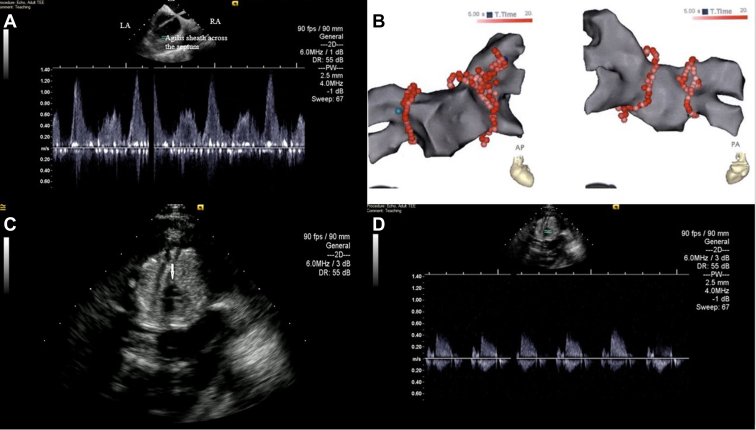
A: Intracardiac echocardiography (ICE) image of Agilis sheath (Abbott) just after transseptal puncture in the plane of left pulmonary veins as evidenced by pulmonary vein pulse vein Doppler images. LA = left atrium; RA = right atrium. B: Pulmonary vein isolation lesion set in anteroposterior (AP) (*left*) and posteroanterior (*right*) views; additional lesions were required to left superior pulmonary vein over the ridge to achieve complete isolation, as seen in AP view. C: ICE imaging of the fetus. From the initial view of the fetus in the long axis, clockwise rotation of the ICE probe revealed a short-axis view with the fetal cardiac atrioventricular (AV) valve (*white arrow*). D: Fetal AV valve Doppler. In the upper half of the image note the pulse wave Doppler cursor at the AV valve. In the lower half of the image note the sweep speed and Doppler for 3 seconds, estimating the fetal heart rate at 120 beats/min.
